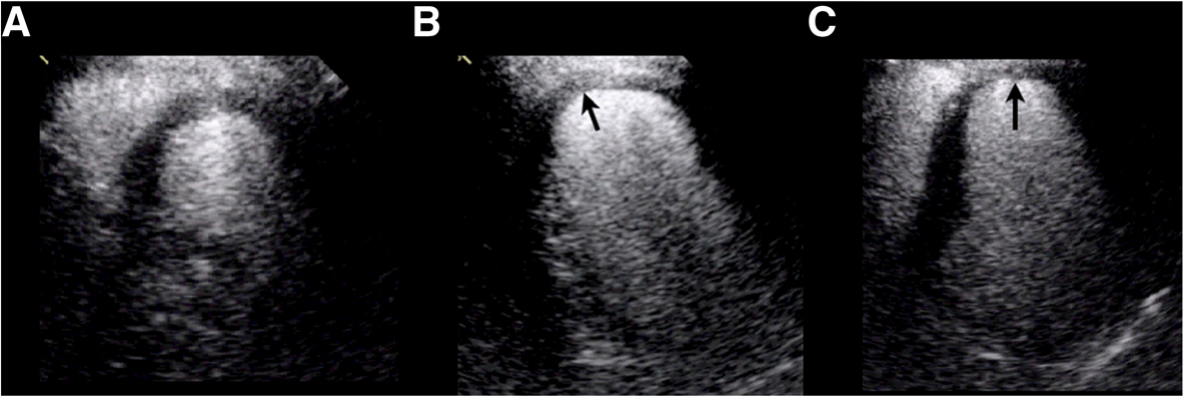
Parasternal short axis view (A), apical 2 chamber view (B), and apical 4 chamber view (C) on transthoracic echocardiography with contrast demonstrating no evidence of an apical thrombus.
